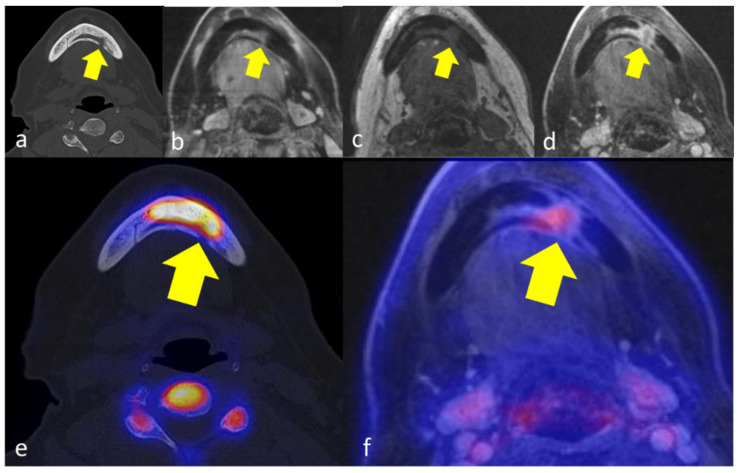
66 years old female patient with secondary chronic OMJ in region 33–35 of the mandible (yellow arrows), showing a diffuse trabecular sclerosis in CT with focal cortical erosion (a), corresponding edema in T2-weighted images (b), and T1-weighted images (c) and increased gadolinium enhancement (d). [18F]fluoride-PET/CT reveals markedly increased tracer uptake in the affected jawbone below the extent of sclerosis (e) and moderately increased tracer uptake in [18F]FDG-PET/MRI (f).
